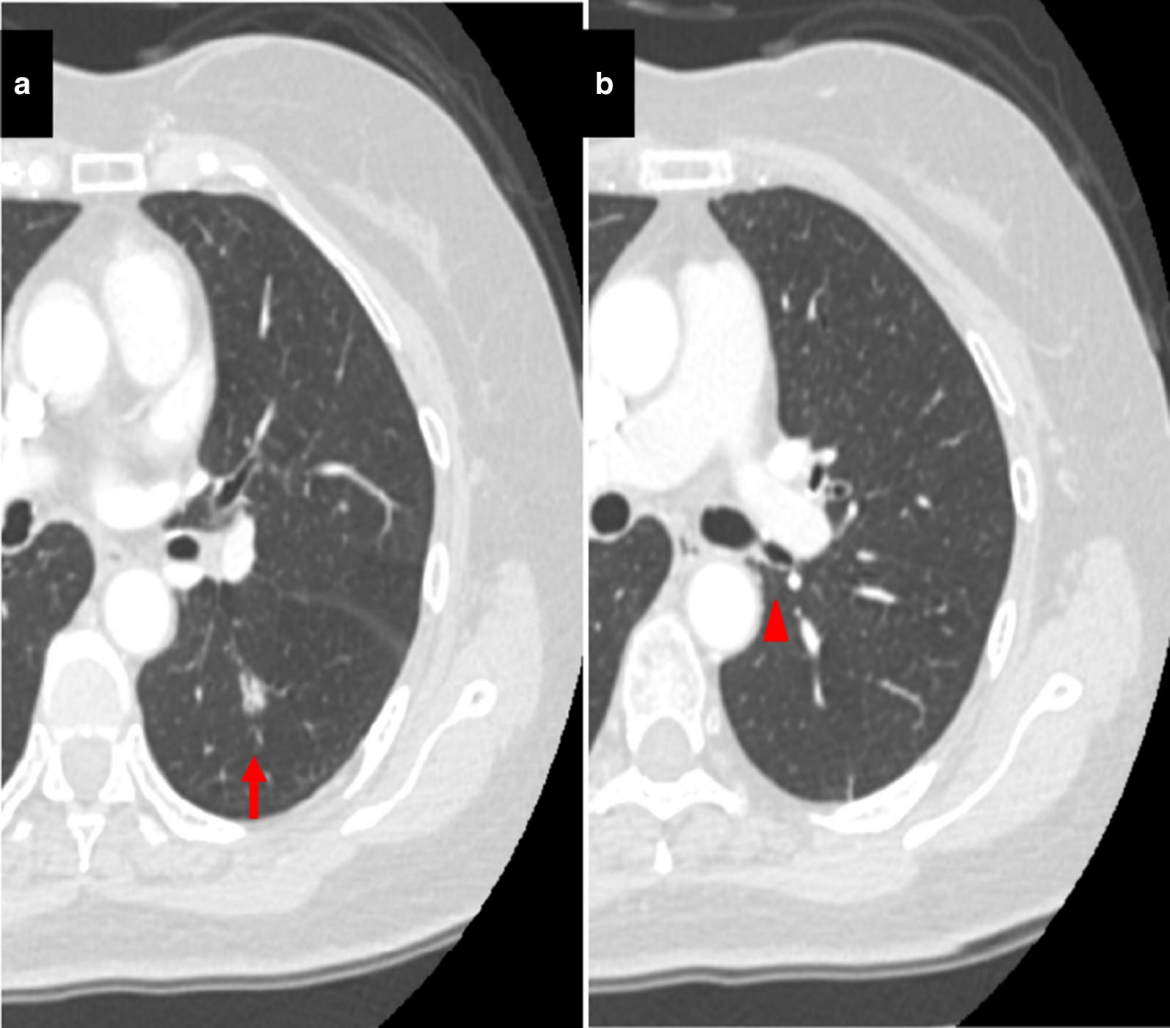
Chest computed tomography images. Contrast-enhanced CT imaging shows the lung tumor located at the left S6 segment (a, arrow) and a displaced B^1+2^ (b, arrowhead)
